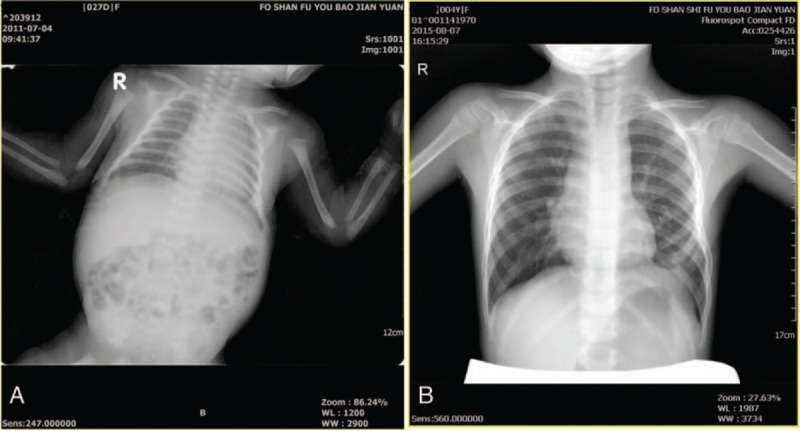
Chest radiographs. (A) At 25 days after birth. (B) At 4 years after birth.
